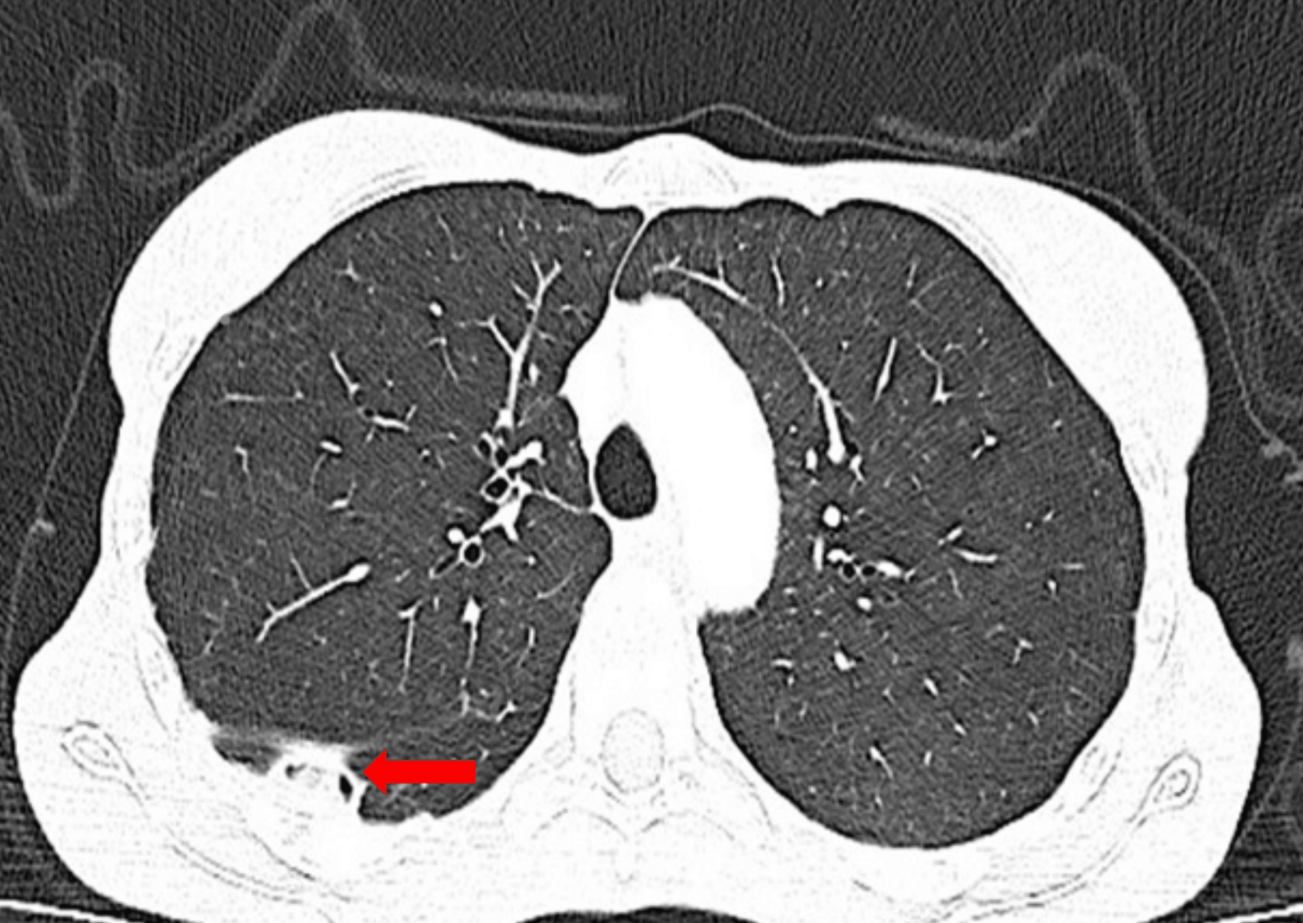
CT thorax showing two cavitating lesions with dependent fluid in the right lung Axial CT thorax (lung window) showing a 2.5 × 1.4 cm cavitary lesion in the superior segment of the right lower lobe (arrow) with dependent fluid (case 1)
